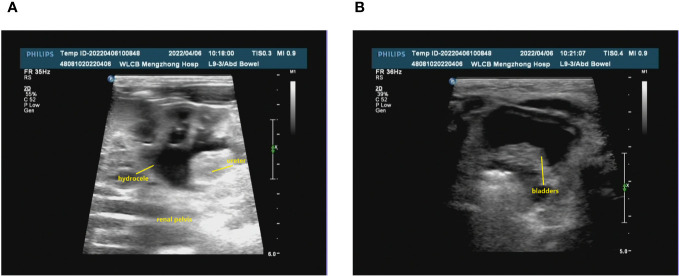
The renal ultrasound of the patient following childbirth. (A) Bilateral kidney assessment: The dimensions of the left kidney were approximately 48x28 mm, exhibiting homogeneous parenchymal echogenicity. No renal echogenicity was observed in the right renal region, and there were no discernible occupying lesions. The renal sinusoidal structure appeared clear, with no evident separation of the right collecting system. The left renal pelvis displayed widening, measuring approximately 9 mm in anteroposterior diameter. Additionally, the left ureter exhibited consistent dilation, reaching a maximum width of approximately 5 mm. (B) The bladder was adequately filled, featuring smooth walls and satisfactory intracapsular translucency.
